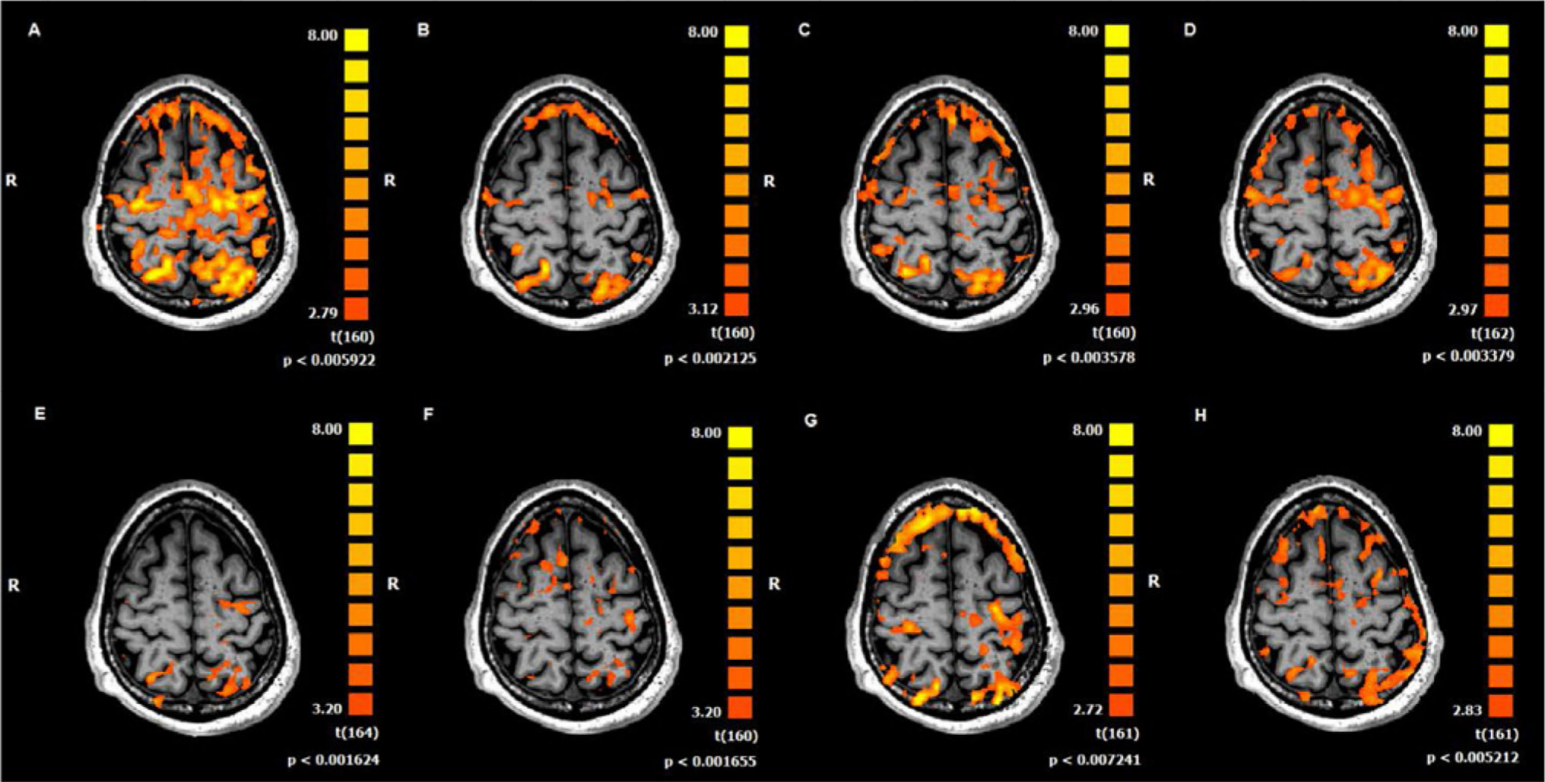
Representative transverse functional magnetic resonance images during paretic hand tracking at (A) Baseline 1, (B) Baseline 2, (C) Posttest 1, and (D) Posttest 2 for the sham rTMS and finger tracking training treatment arm. (E–H) represent the same time points for images taken from the active rTMS and finger tracking training treatment arm. For all images FDR <0.02 and Talairach coordinate z = 55.
